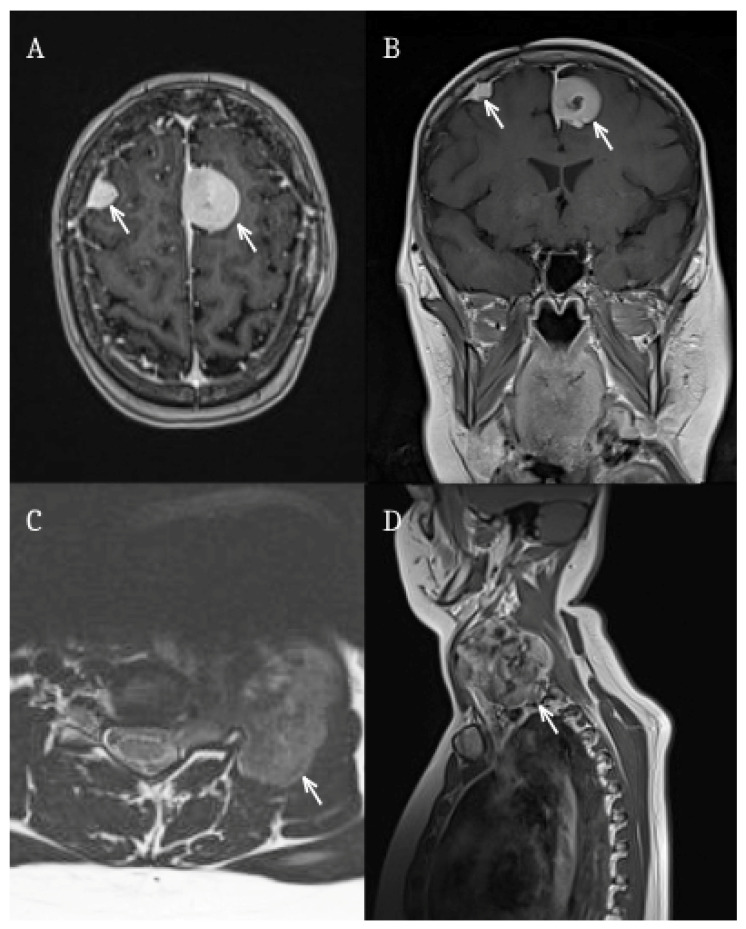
Magnetic resonance imaging findings in Patient 2 with RIMs. White arrows indicate the tumors. (A) Axial T1-weighted post-contrast image demonstrating multifocal intracranial meningiomas. (B) Coronal T1-weighted post-contrast image confirming multiple contrast-enhancing lesions. (C) Axial MRI of the cervical region showing a large extracranial mass suspected to represent a meningioma. (D) Sagittal MRI of the cervical spine demonstrating the extent of the cervical lesion.
